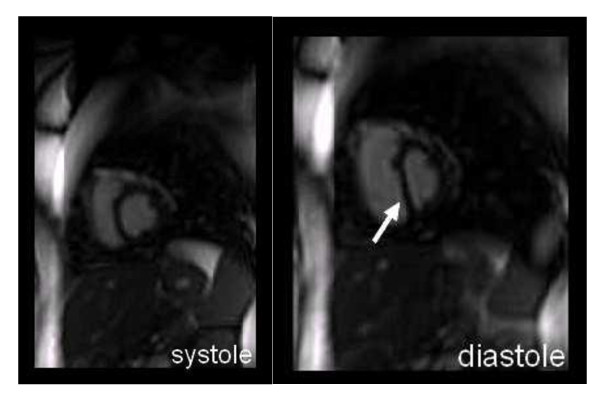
short axis MRI showing early diastolic flattening of the interventricular septum.
